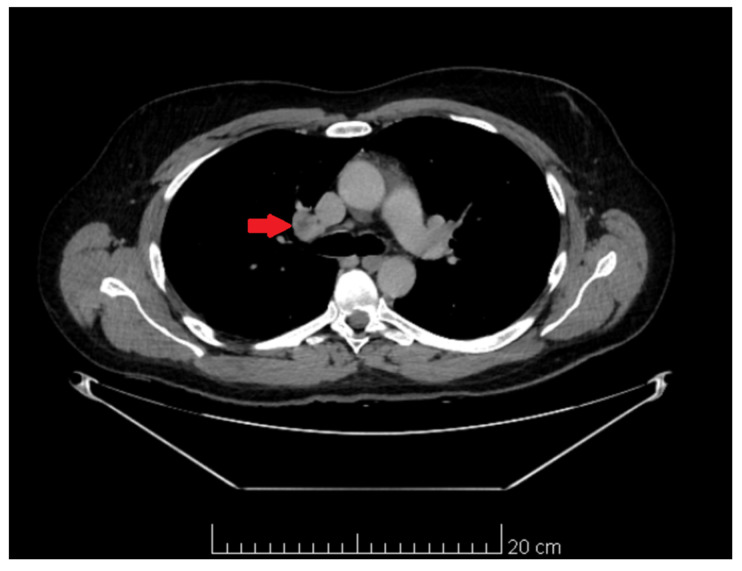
CT image. Pathological lymph node with central necrosis in the hilum of the right lung.
